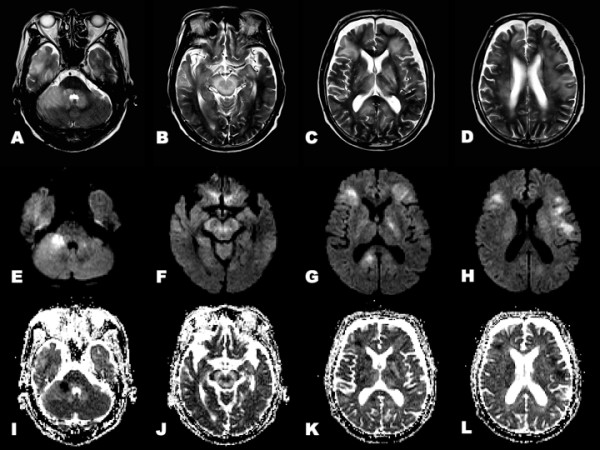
Brain MRI after neurological worsening. A-D. T2-weighted image show widespread T2-hyperintense lesions in the brainstem, cerebellum, basal ganglia and cerebral white matter. E-H. DWI shows hyperintense lesions in the middle cerebellar peduncles, midbrain, left internal capsules and cerebral white matter. I-L. ADC shows hypointense lesions in the middle cerebellar peduncles, midbrain, left internal capsules and cerebral white matter.
